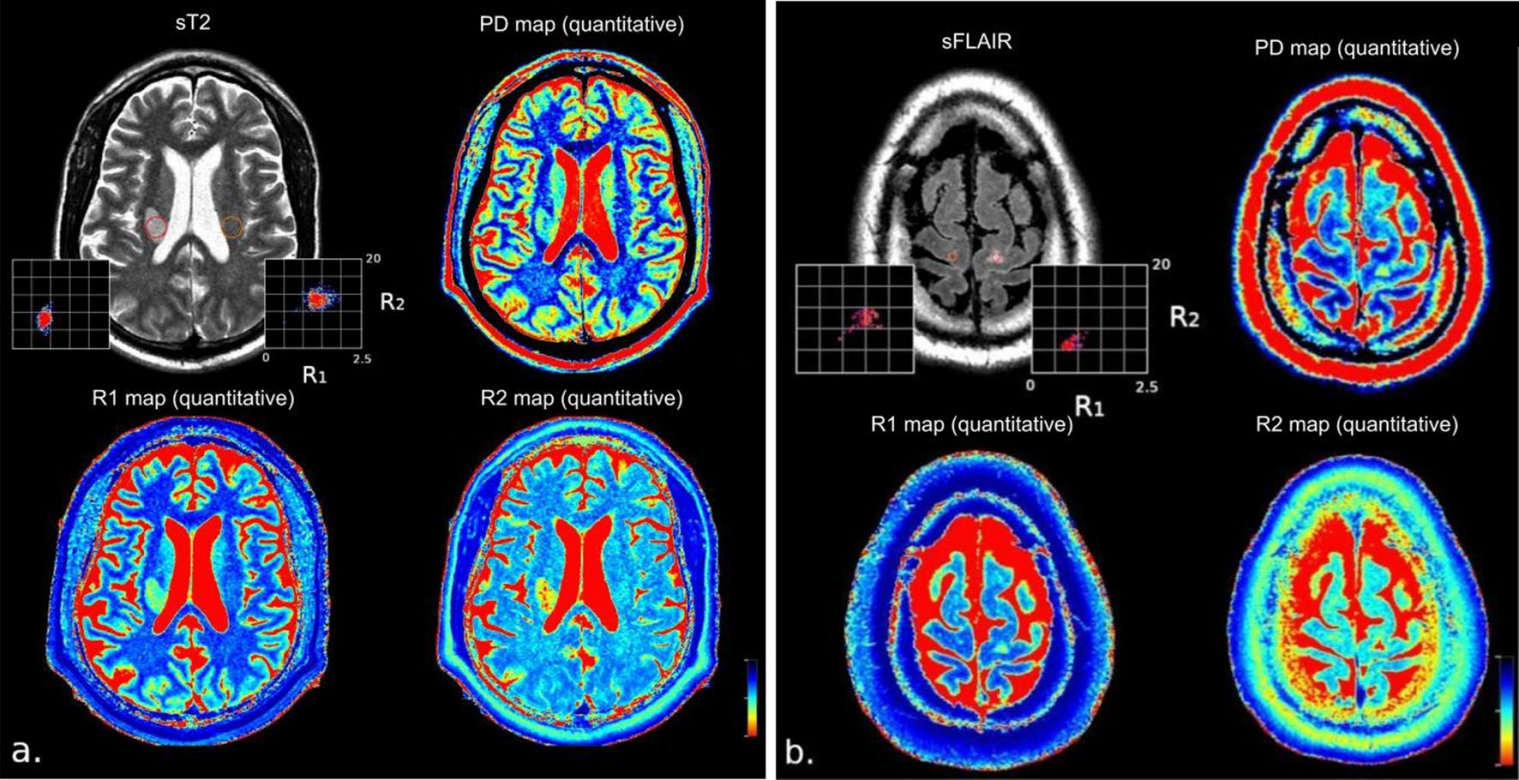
Representative examples of ROI in lesions (a acute ischemic lesion DWI + /rADC, b chronic ischemic lesion WMH) and contralateral mirror NBR in a sT2 weighted image (upper left quadrant) with R_1_R_2_ Plot (scales: 0–2.5 [1/s]; 0–20 [1/s]) and R1 (lower left quadrant, red-to-blue colorbar scale: 0–2000 [ms]), PD (upper right quadrant, red-to-blue colorbar scale: 0–100 [pu]) and R2 (lower right quadrant, red-to-blue colorbar scale: 0–200 [ms]) quantitative maps.
